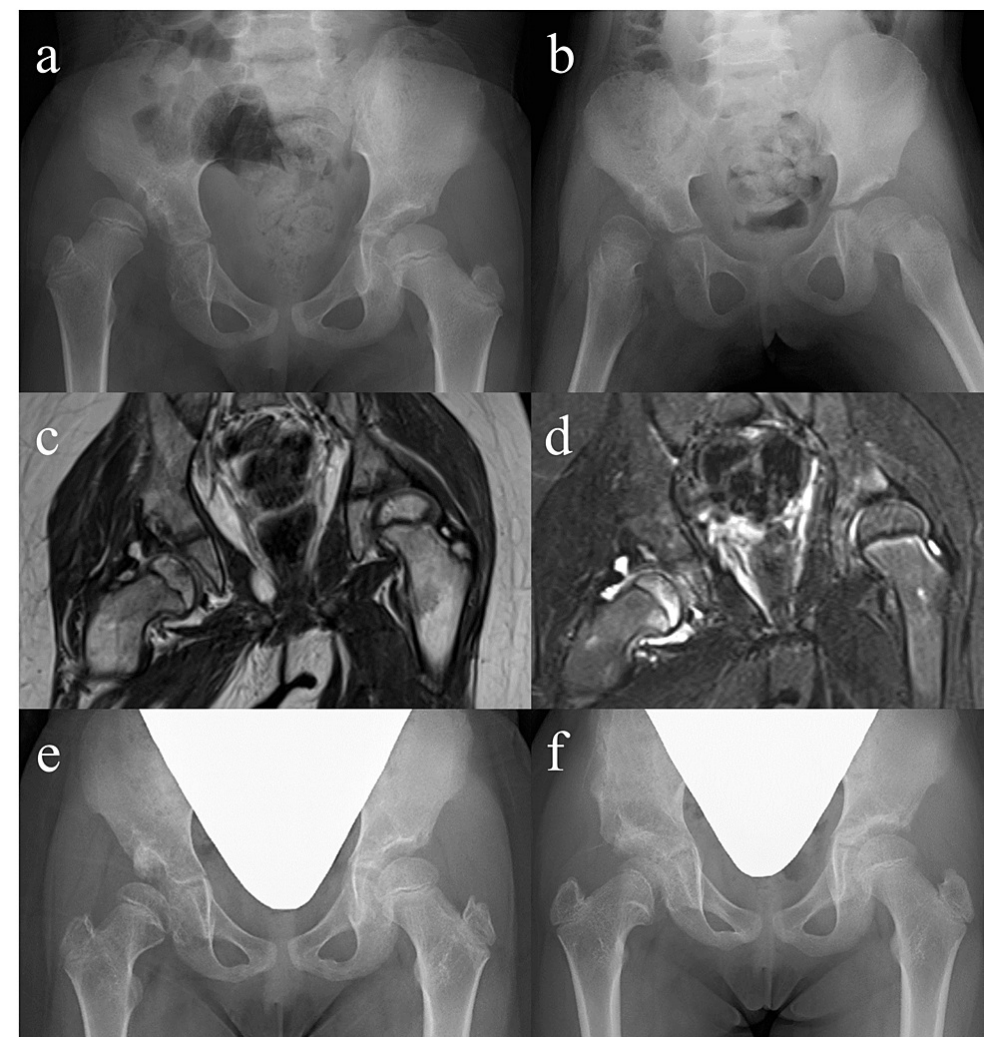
Supine anteroposterior hip radiographs and MRI images of Case 3. (a) An initial hip radiograph obtained at five years and 11 months of age showing a right-sided developmental dysplasia of the hip with Yamamuro’s “distance a” of −10 mm. (b) A follow-up hip radiograph obtained after 48 days of horizontal skin traction showing a decent “distance a” of −1 mm. (c and d) T2-weighted coronal (c) and short-tau inversion recovery coronal (d) MRI images obtained at the initiation of treatment with a ﬂexion-abduction brace demonstrating intracapsular effusions and edematous changes involving the medial portion of the capital femoral epiphysis and metaphysis. (e) A follow-up hip radiograph obtained after treatment with a ﬂexion-abduction brace showing the residual acetabular dysplasia of the right hip. (f) The latest hip radiograph obtained two and a half years after Salter innominate osteotomy demonstrating an obvious coxa vara with a shortened femoral neck and a slightly aspherical femoral head. Note a thin osteosclerotic line at the medial aspect of the femoral neck, possibly representing transient growth arrest after reduction.
